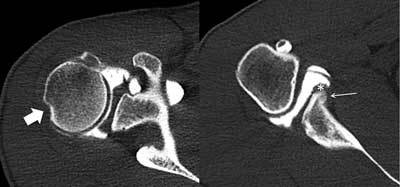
CT arthrography of the right shoulder in a 24-year-old male patient with anteroinferior shoulder instability demonstrating a Hill-Sachs defect (broad arrow on left image) posterosuperior on the humeral head on a transverse image. More caudally (right image) a scarred and medialized labrum can be found (asterisk) adjacent to a healed anterior glenoid rim fracture with residual callus (thin arrow).
